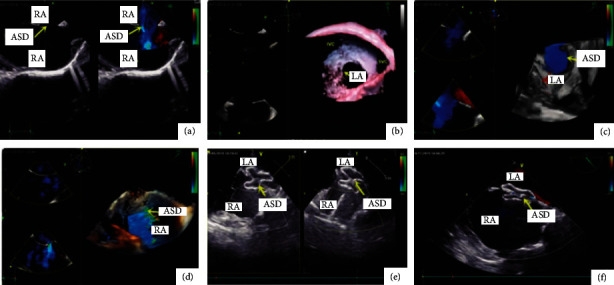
Ultrasound evaluation of the diaphragm image (some components in the picture come from the internet and have been authorized by the author).
